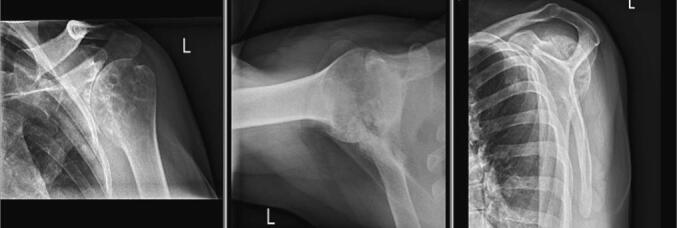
Preoperative radiographic images of left shoulder showing degenerative joint disease in patient II.
